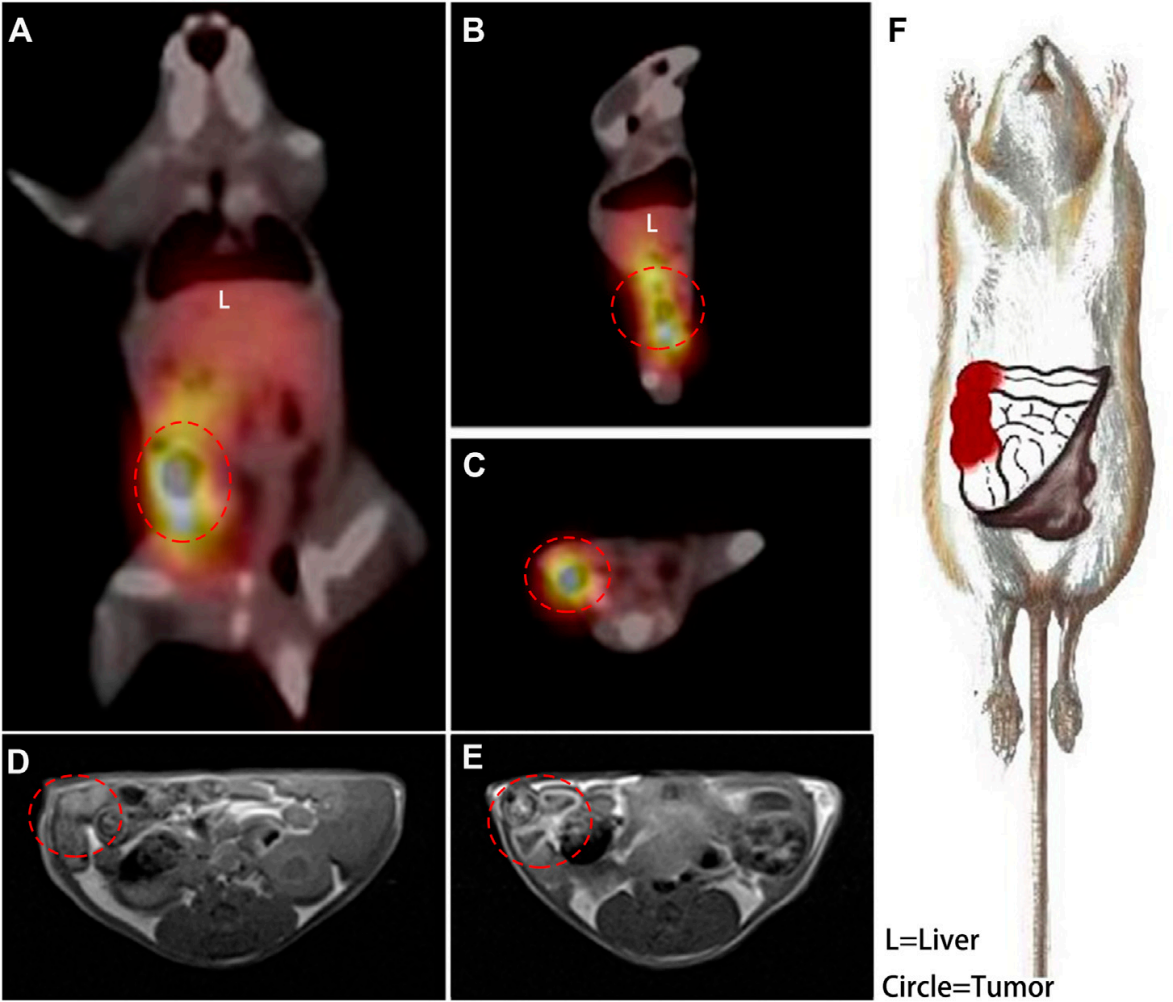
A coronal plane (A), sagittal plane (B) and cross-section (C) SPECT images acquired at 6 h post injection of ^99m^Tc-DTPA-nivolumab, and the right colon with high tracer uptake is circled. In transverse T_1_WI (D), the suspected thickened intestine is circled, and the enhanced intestinal wall is circled in an enhanced scan acquired at 2 h post injection of Gd-DTPA-nivolumab (E). A detailed description of lesions with high PD-1 (in red) was drawn based on these functional imaging findings (F).
